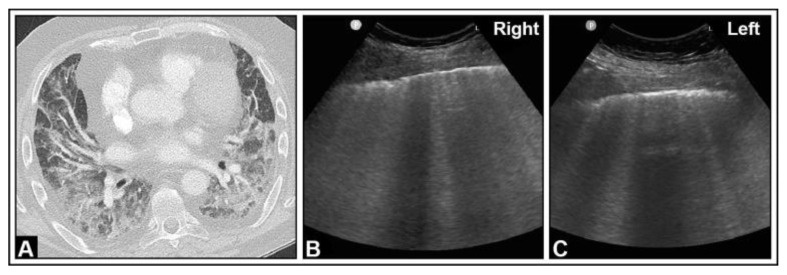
A patient with granulomatosis with polyangiitis undergoing rituximab and cyclophosphamide therapy, currently presenting with COVID-19 pneumonia and severe acute respiratory distress syndrome according to the Horovitz Index. (A) In the chest computed tomography, areas with lung consolidations and ground-glass opacities are present bilaterally. (B,C) In the B-mode ultrasound, multiple B-lines can be seen in both the right lung and the left lung.
